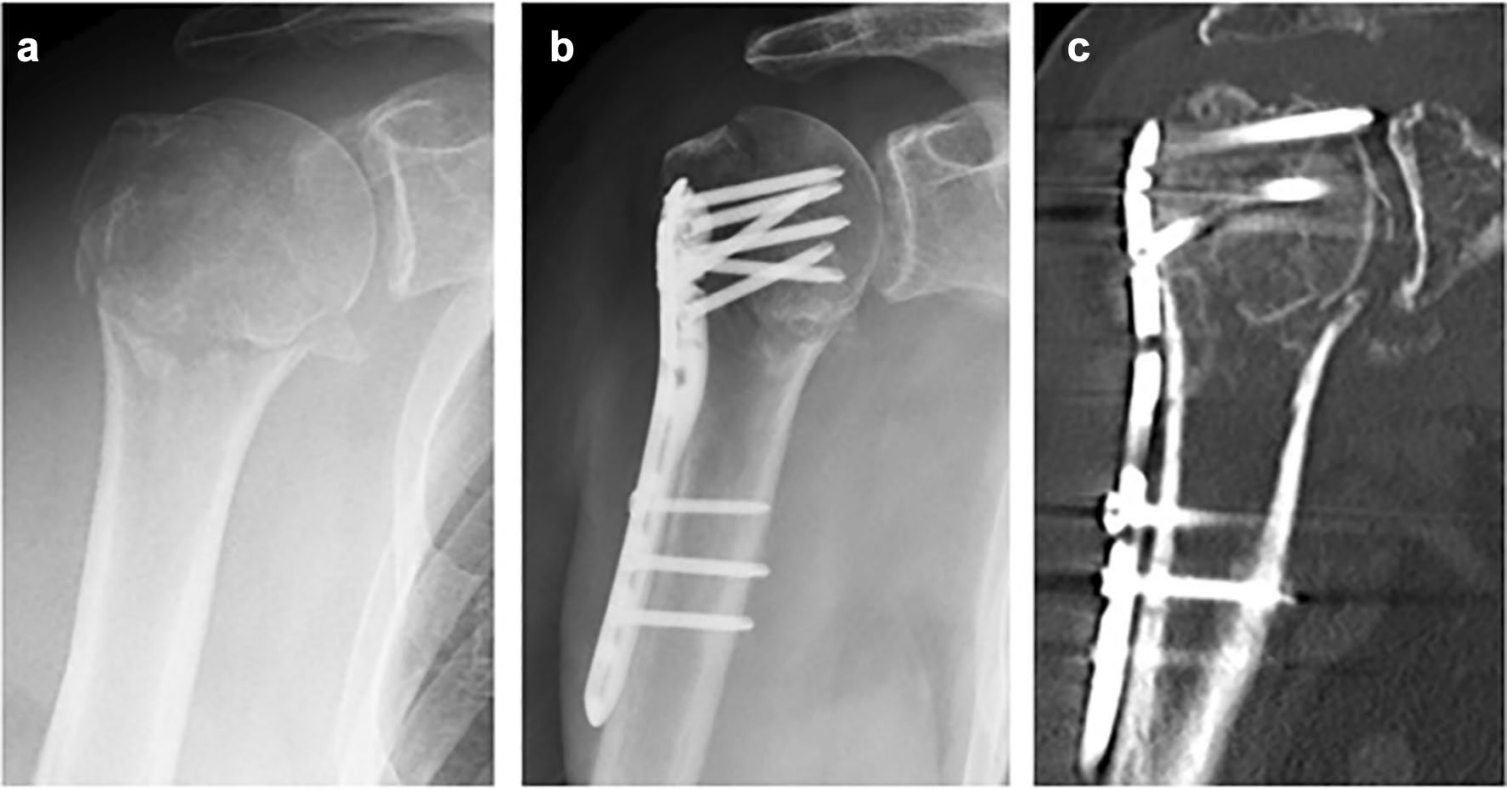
Case presentation. A 74 year old woman presented with a four-part fracture of the right humeral head. Open reduction and internal fixation was performed 1 day after the trauma. a preoperative ap-view of the fracture; b postoperative ap-view after reconstruction using a locking plate osteosynthesis; c CT scan two months postoperative shows a collapse of the humeral head with secondary dislocation of the screws
